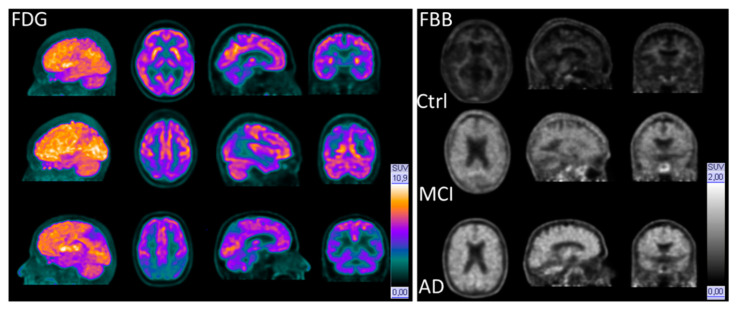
Exemplary 3D, transversal, sagittal, and coronal PET images of healthy control, MCI, and AD (top to down) using [^18^F]FDG (left) and [^18^F]FBB (right).
